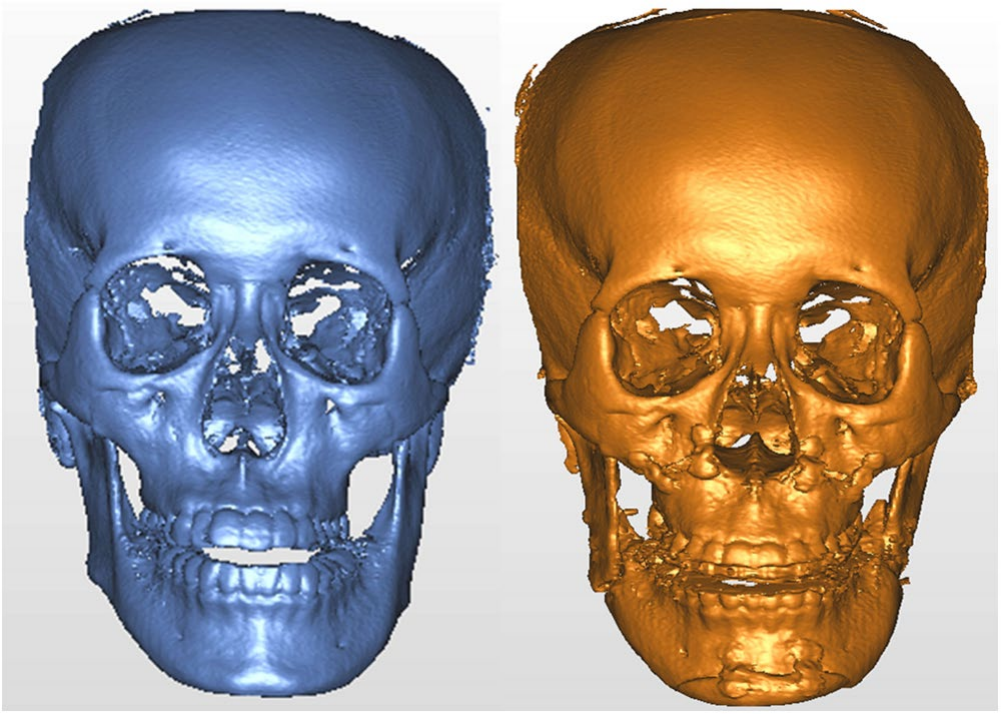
(Left) Preoperative and (right) postoperative cone-beam computed tomography-based craniofacial images of same patient as in Fig. 1. Postoperative measurement of the rotation showed 4.5° and 3.8° in pitch, 3.6° and 7.4° in roll, and 4.4° and 4.9° in yaw on her right and left planes of proximal segment (PSPs), respectively.
